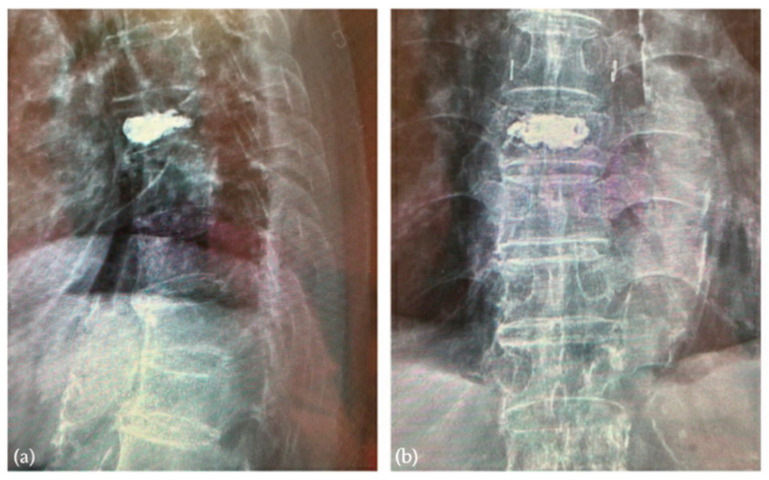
Postoperative radiographs showing no evidence of cement leakage. (a) Lateral view. (b) Anteroposterior view.
